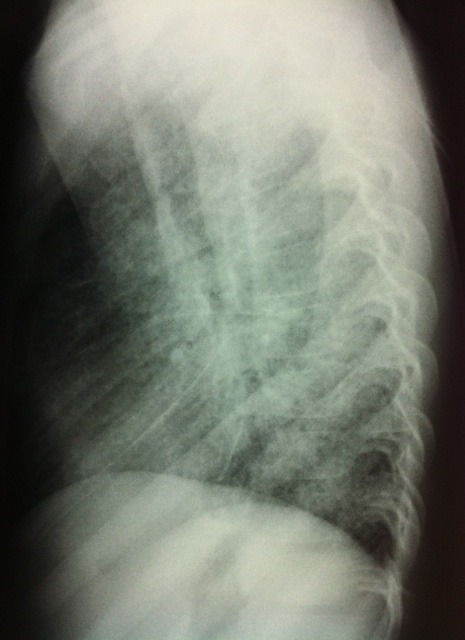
Lateral chest X-rays showing bilateral diffuse nodular infiltrates, similar to miliary TB
